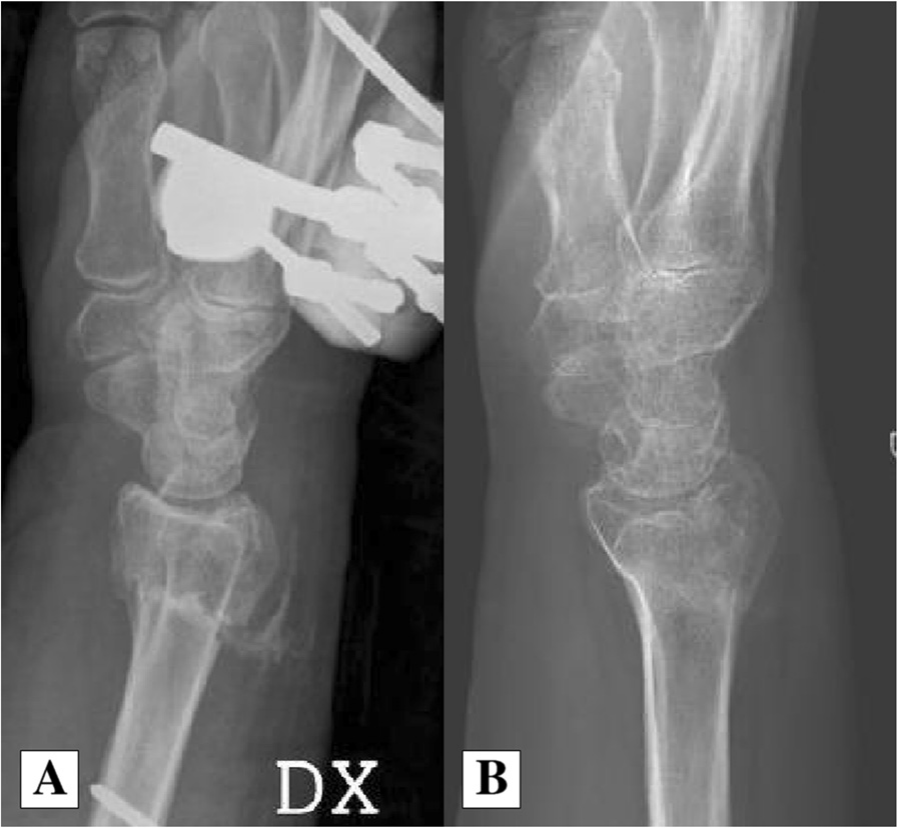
Postoperative radiograph and 6 months after. Postoperative radiograph after external fixation and dorsal injection of Augment® into the fracture gap (A). At 6 months, all Augment® has resolved (B).
